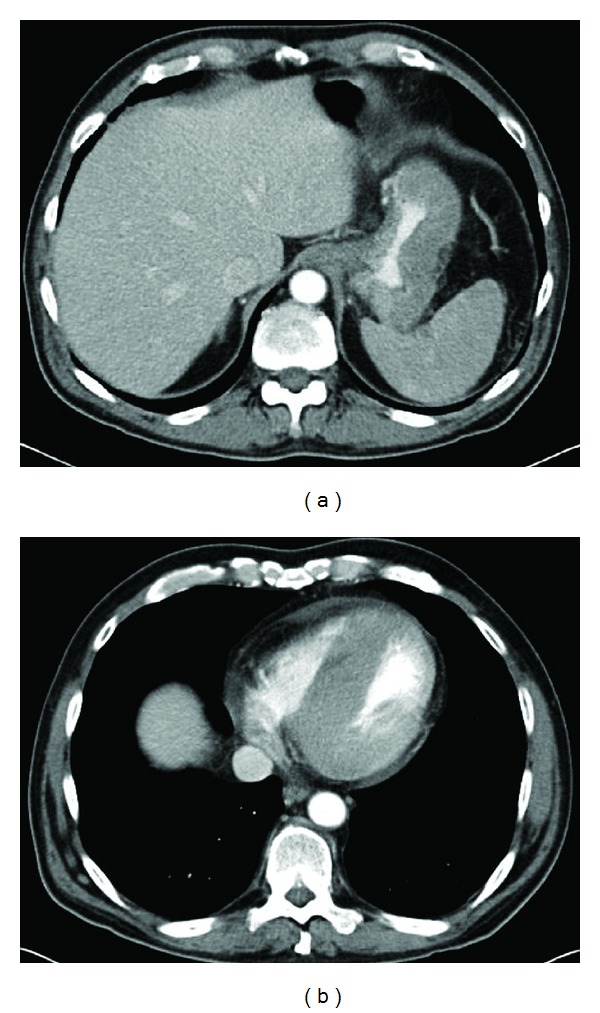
Computed tomography showing diffuse thickening of the gastric wall (a) and interventricular septum (b) due to infiltration of myeloid sarcoma.
